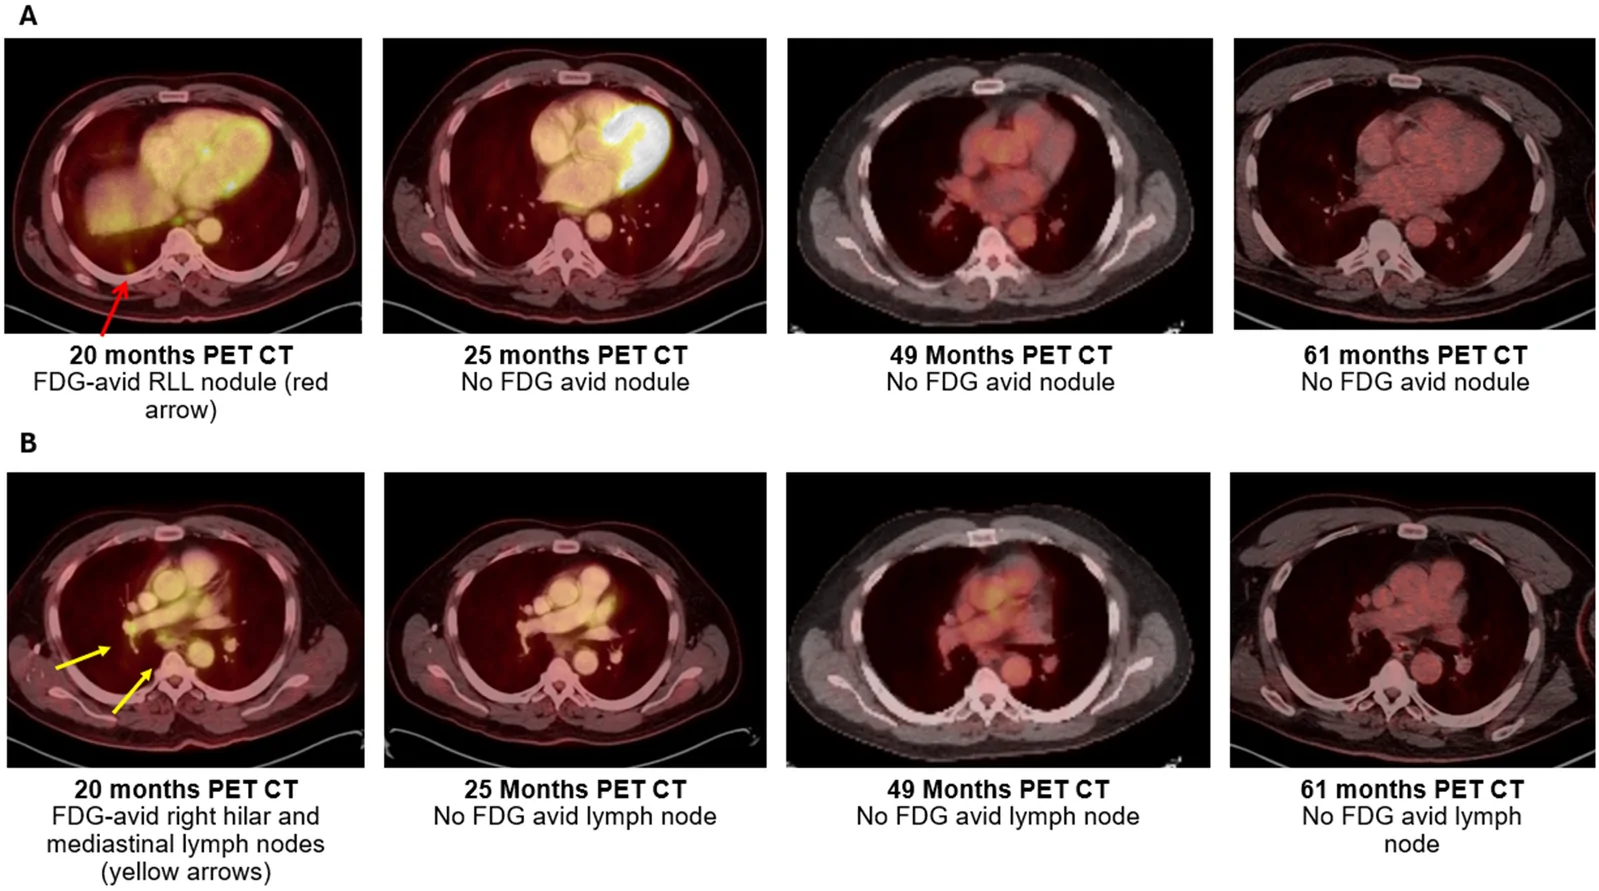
PET CT radiological imaging over time. (A) Presentation and resolution of an FDG-avid RLL nodule over the treatment period. (B) Presentation and resolution of FDG-avid right hilar and mediastinal lymph nodes during alectinib treatment.
